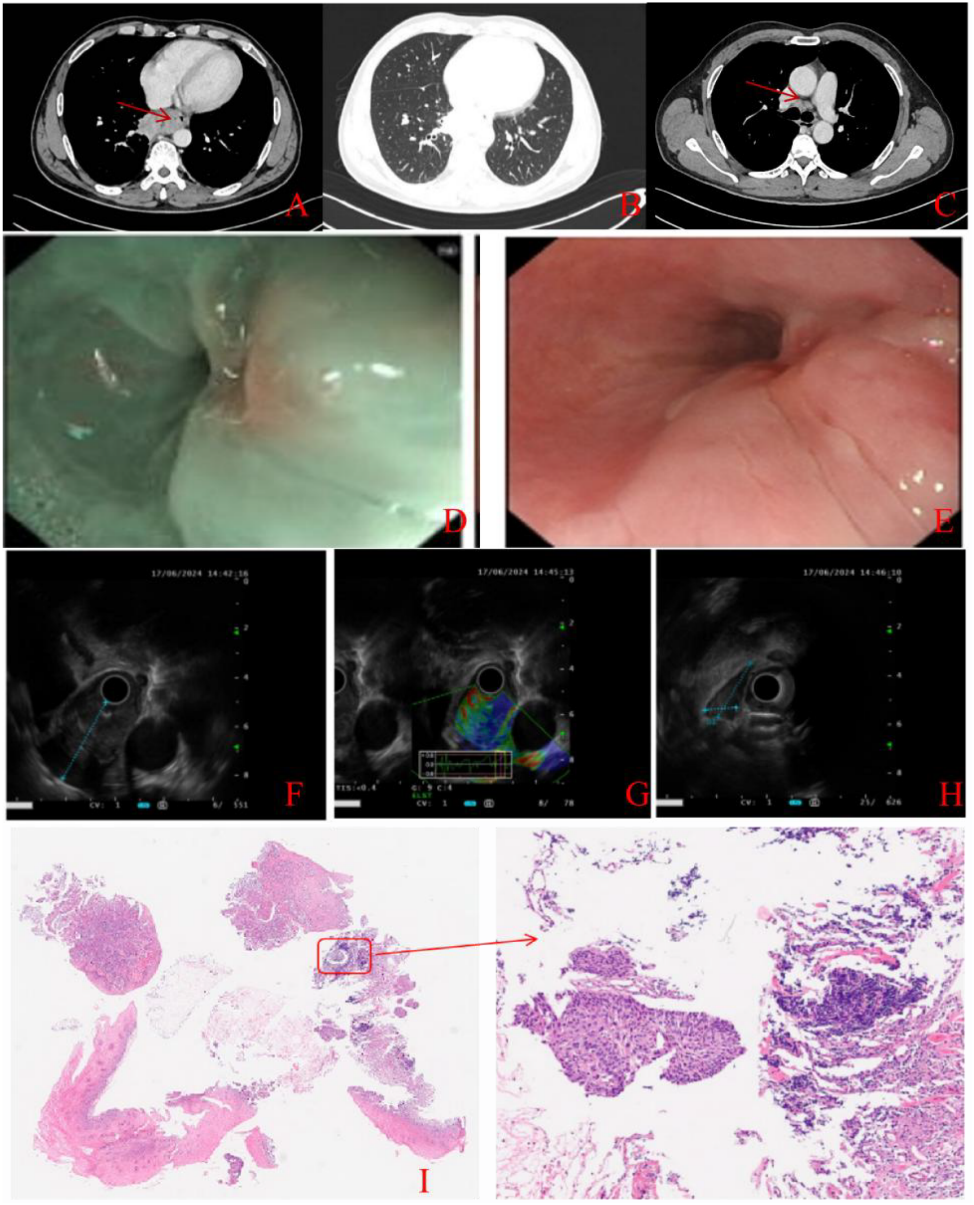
Imaging examination before patient treatment. (A-C) CT image on June 12, 2024, (A) shows the primary lesion (arrow indicates the primary tumor), (B) showing the lung window and (C) showing the mediastinal lymph nodes (arrow indicates the mediastinal lymph nodes, station 4R). (D, E) Upper gastrointestinal endoscopy. (F-H) Ultrasound gastroscopy images at diagnosis, with (F) showing the Ultrasound of lesion, (G) showing the Elastrography. (H) showing the Mediastinal ultrasound. (I) Pathological image at diagnosis.
